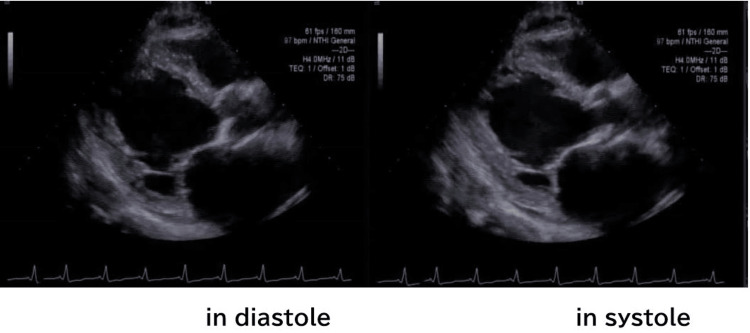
Echocardiography showing left ventricular systolic dysfunction The LVEF was 20%, and the LAD was 58 mm (normal: 30-35 mm). The LVDd was 69 mm (normal: 41-47 mm), and the LVDs were 63 mm (normal: 18-32 mm). The myocardial hypertrophy was mild and the IVSTd was 11 mm (normal: 7-9 mm). The LVPWd was 12 mm (normal: 6-10 mm). Tethered mitral valve and severe mitral regurgitation were noted. LVEF: Left ventricle ejection fraction, LAD: Left atrial dimension, LVDd: Left ventricular internal dimension in diastole, LVDs: Left ventricular internal dimension in systole, IVSTd: Intraventricular septum thickness at end-diastole, LVPWd: Left ventricular posterior wall thickness at end-diastole
